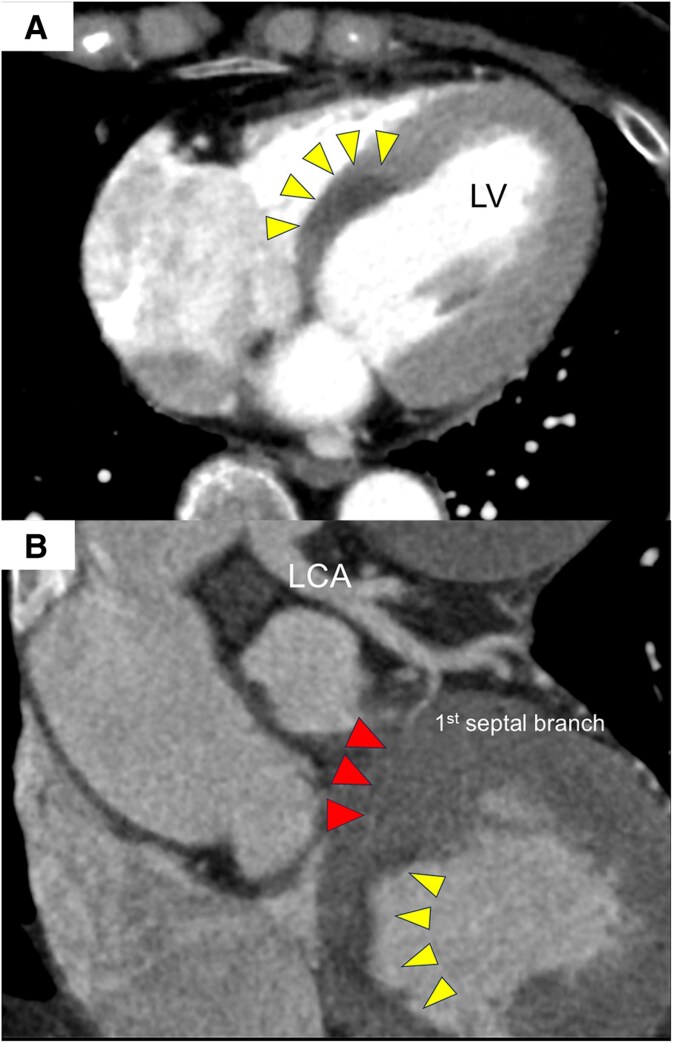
Contrast-enhanced computed tomography findings. (*A*) A contrast-impaired area is seen at the base of the ventricular septum in the arterial phase (yellow arrowheads). (*B*) Stenosis of the perfused septal branch is observed in the low-contrast area (red arrowhead). LCA, left coronary artery; LV, left ventricular.
